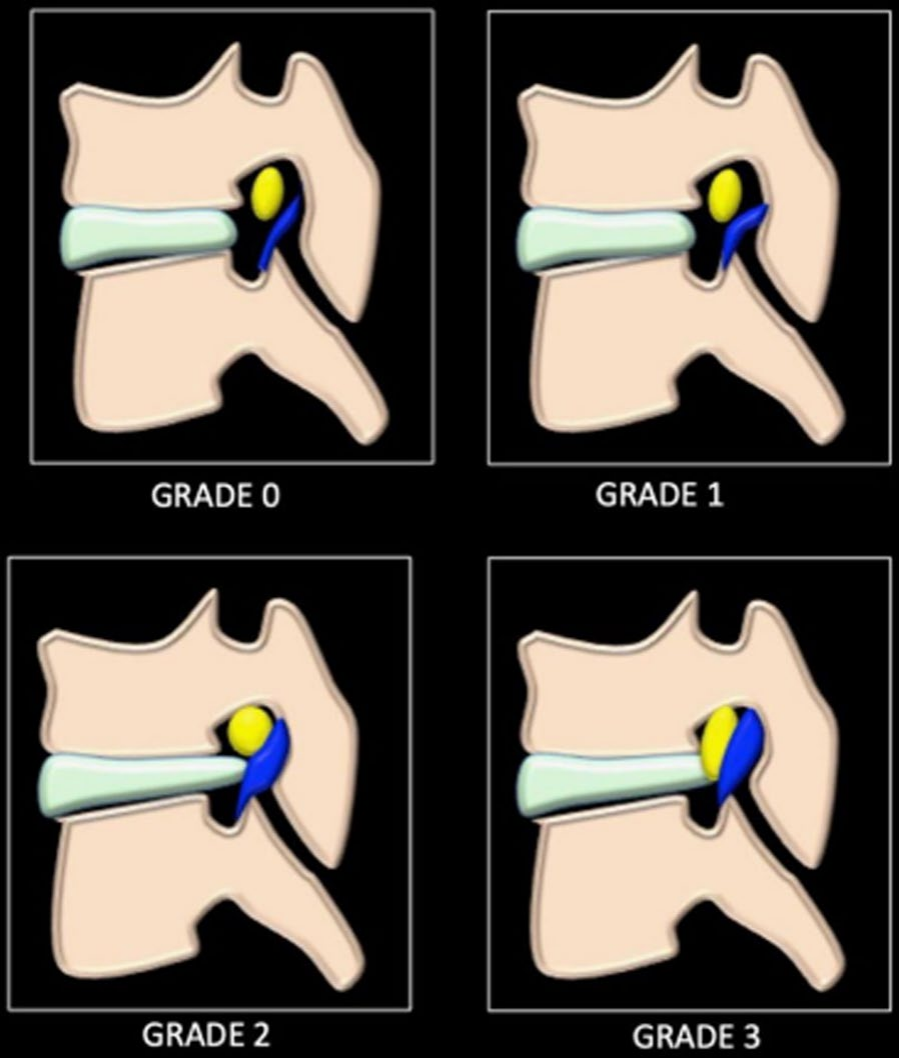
CT-based classification system of lumbar foraminal stenosis, normal foramen—grade 0, anteroposterior/superior–inferior fat compression—grade 1, both anteroposterior/superior–inferior compressions with no distortion of nerve root—grade 2, and grade 2 with additional distortion of nerve root—grade 3 [17]
